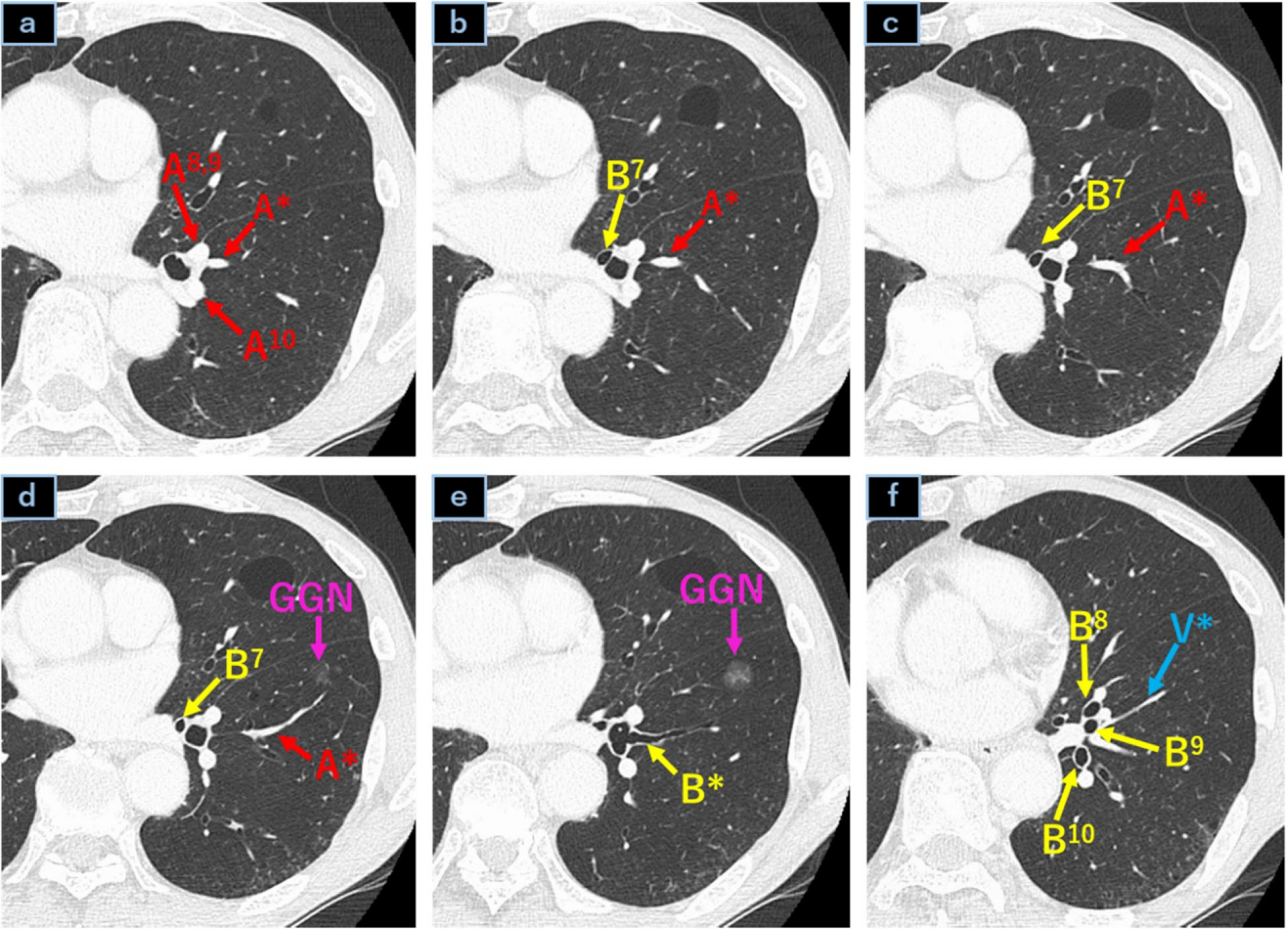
a–f Chest computed tomography images. A subsuperior segmental bronchus (B*) and B7 were detected by thin section computed tomography, and a ground-glass nodule (GGN) was detected in the subsuperior (S*) segment. The subsuperior segmental vein (V*) was included in the S*
